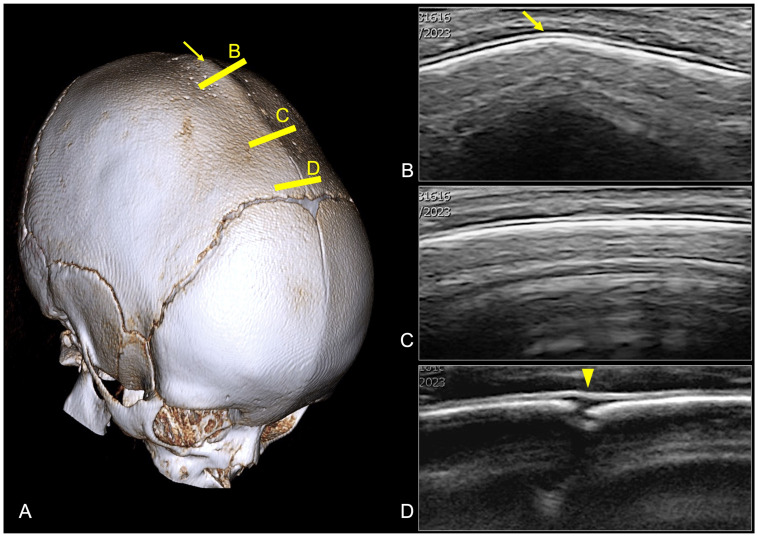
Premature fusion of the sagittal suture in a 12-month-old boy. (A) CT volume rendering showing the dolichocephalic shape of the head, with complete fusion of the sagittal suture and sagittal ridge on the medial aspect of the suture. (B,C) US images obtained at the level of the yellow lines positioned on the 3D CT image showing the fused sagittal suture as a continuous hyperechoic calvaria with loss of the expected hypoechoic gap and the presence of a sagittal ridge (yellow arrow). (D) A small portion of the patient’s sagittal suture is shown on the very anterior aspect of the suture close to the anterior fontanelle as a hypoechoic gap (yellow arrowhead).
